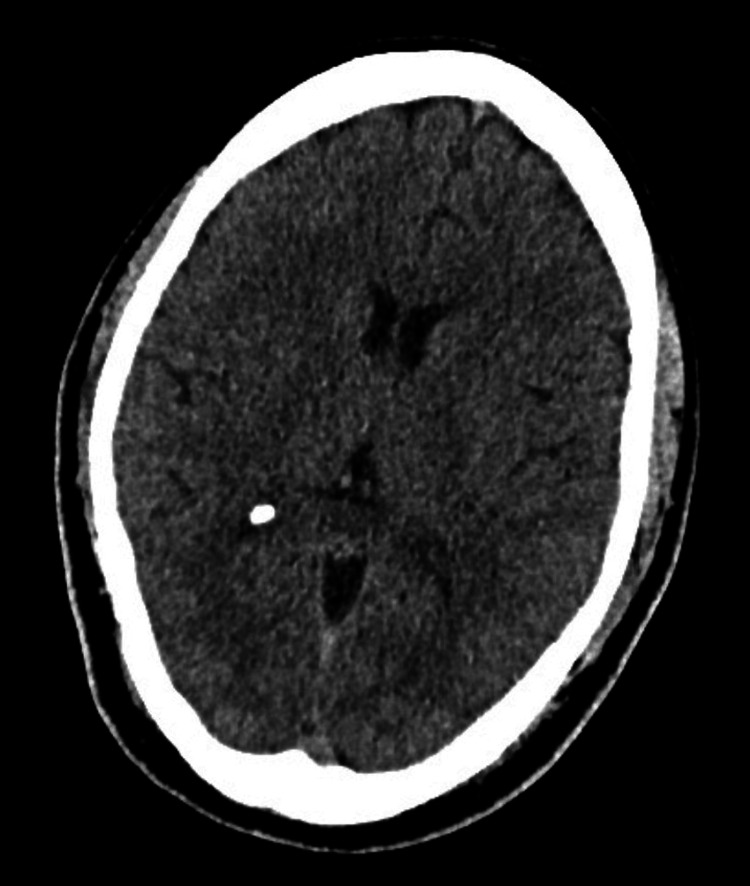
Non-contrast CT scan of the head Non-contrast CT scan of the head showing no evidence of acute intracranial pathology. Ventricular size and sulcal pattern are within normal limits, with no signs of haemorrhage, mass effect, or midline shift.
